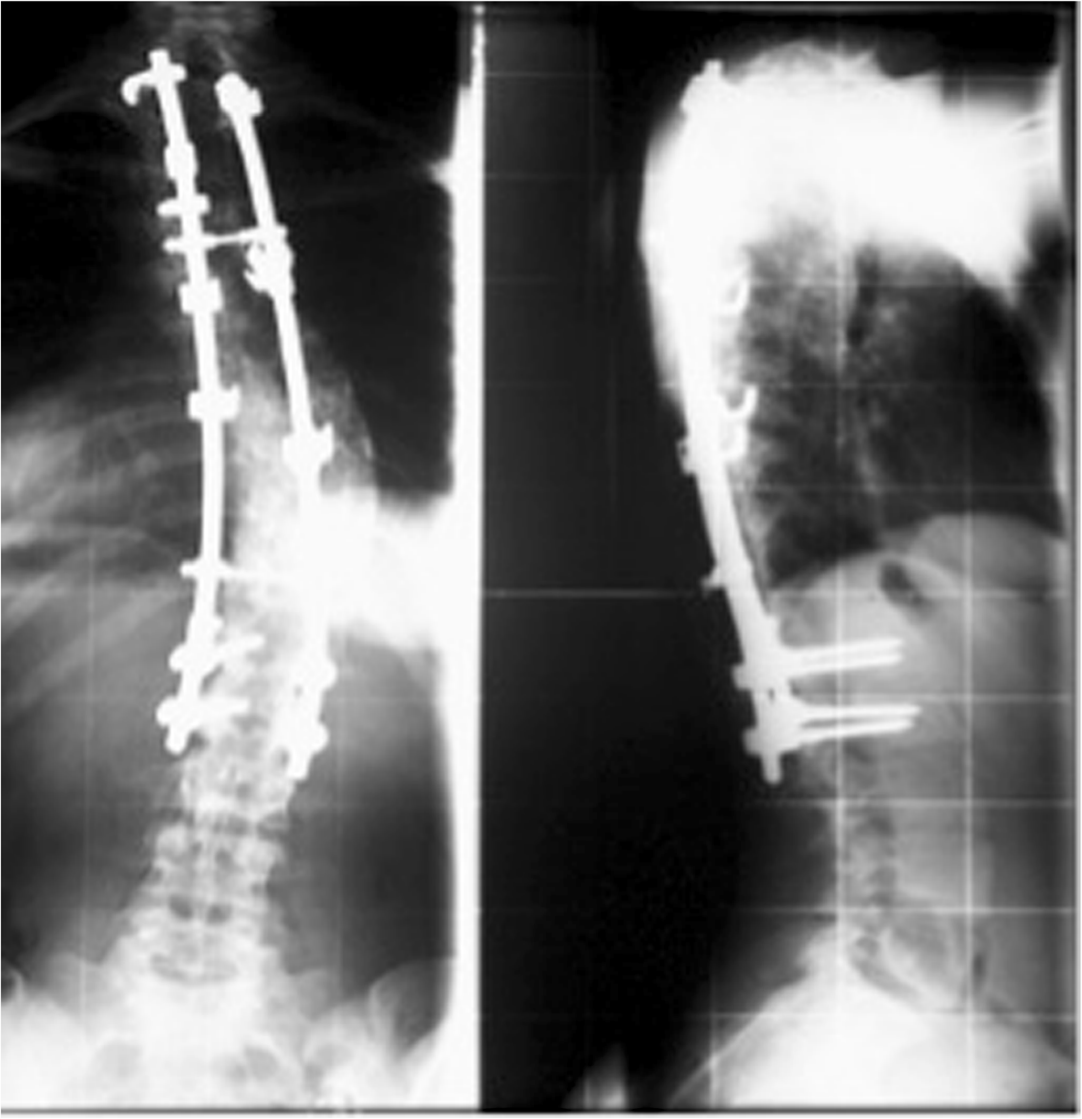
X-rays of CD instrumentation with hooks and pedicle screws (so-called hybrid technique).
